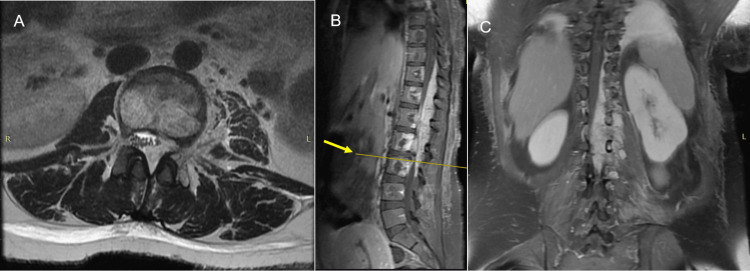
MRI showing thoracolumbar extension (A) Axial T2-weighted MRI at L3 level showing the displacement of the nerve roots of the cauda equina. (B) Sagittal T1-weighted MRI showing the hyperintense behavior of the lesion (arrow and line indicate L3 level). (C) Same-level coronal T1-weighted MRI using gadolinium-based contrast agent showing the left predominance of the lesion with extension toward the ipsilateral neuroforamen MRI: magnetic resonance imaging
